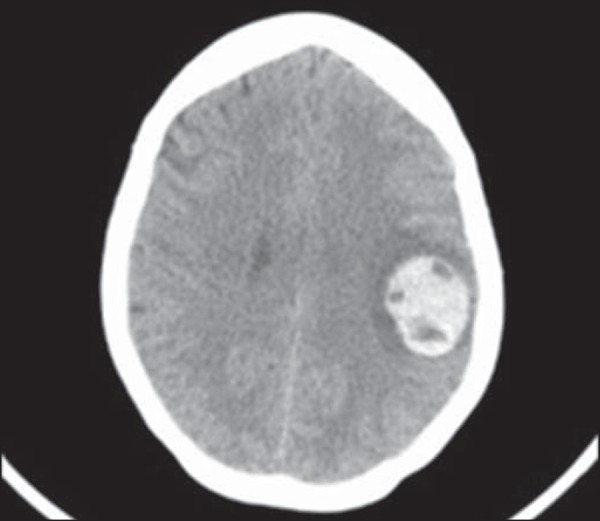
MRI scan of the brain, showing a mass suggestive of metastatic
